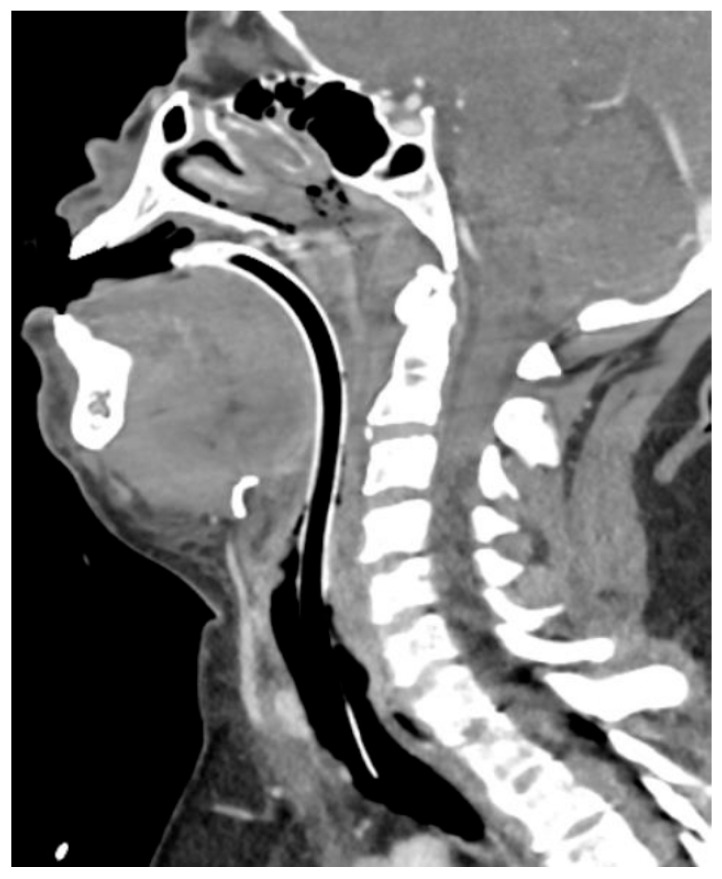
Sagittal image from a computed tomography scan of the neck with intravenous contrast, demonstrating a collapsed hypopharyngeal and laryngeal airway due to surrounding tissue edema, with endotracheal tube in situ securing the airway.
